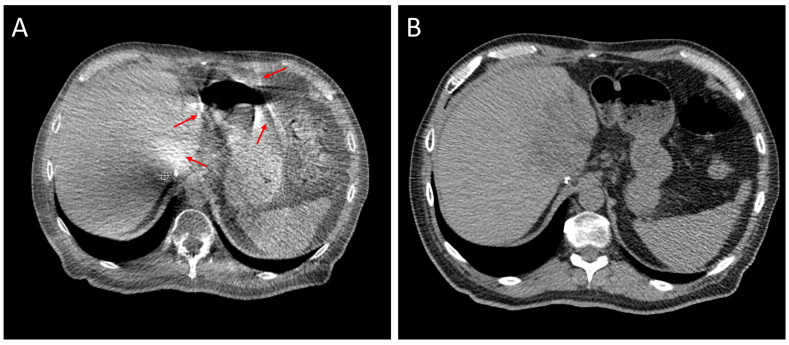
A comparison between (A) a BH CBCT image and (B) a BH CT-on-rails image of the same patient and anatomical location. Note the artifacts (red arrows) near the gas and high-density object in the CBCT image, which are not shown in the CTOR image. The boundaries of the stomach and bowel are also more clearly visualized in the CTOR image, compared to the CBCT image. Also visible in the CTOR image, are features within the liver itself.
